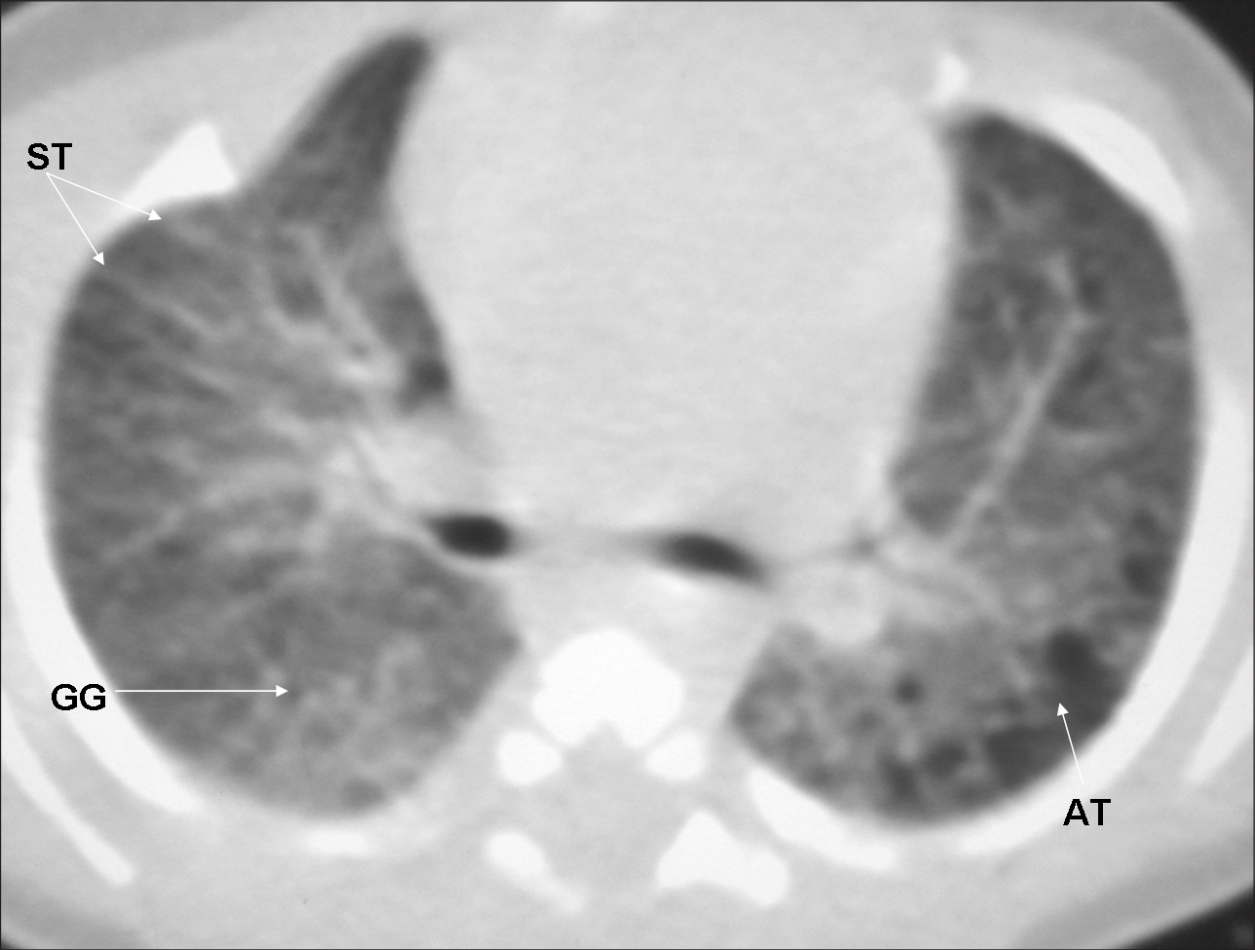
High-resolution computed tomography (HRCT) on a very low birth weight premature infant, showing air trapping (AT), ground glass opacity (GG) and septal thickening (ST).
